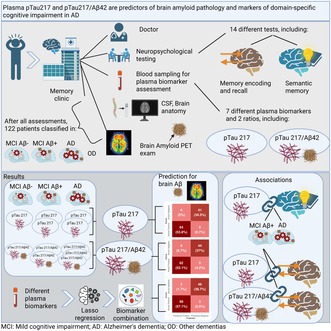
AbbreviationsADAlzheimer's diseaseAPOEapolipoprotein EAUCarea under curveAβamyloid betaCSFcerebrospinal fluidCTcomputed tomographyFDGfluorodeoxyglucoseFDRfalse discovery rateGFAPGlial fibrillary acidic proteinInDaPCAprincipal component analysis for incomplete data handlingLASSOleast absolute shrinkage and selection operatorLOOCVcross‐validation with the leave‐one‐out methodMCImild cognitive impairmentMMSEMini‐Mental State ExaminationMRImagnetic resonance imagingNFLneurofilament light chainNPneuropsychologicalNPVnegative predictive valuePCprincipal componentPCAprincipal component analysisPETpositron emission tomographyPPVpositive predictive valueRAVLTRey auditory verbal learning testROCreceiver operating characteristicROCFTRey‐Osterrieth complex figure testVRvisual reads
